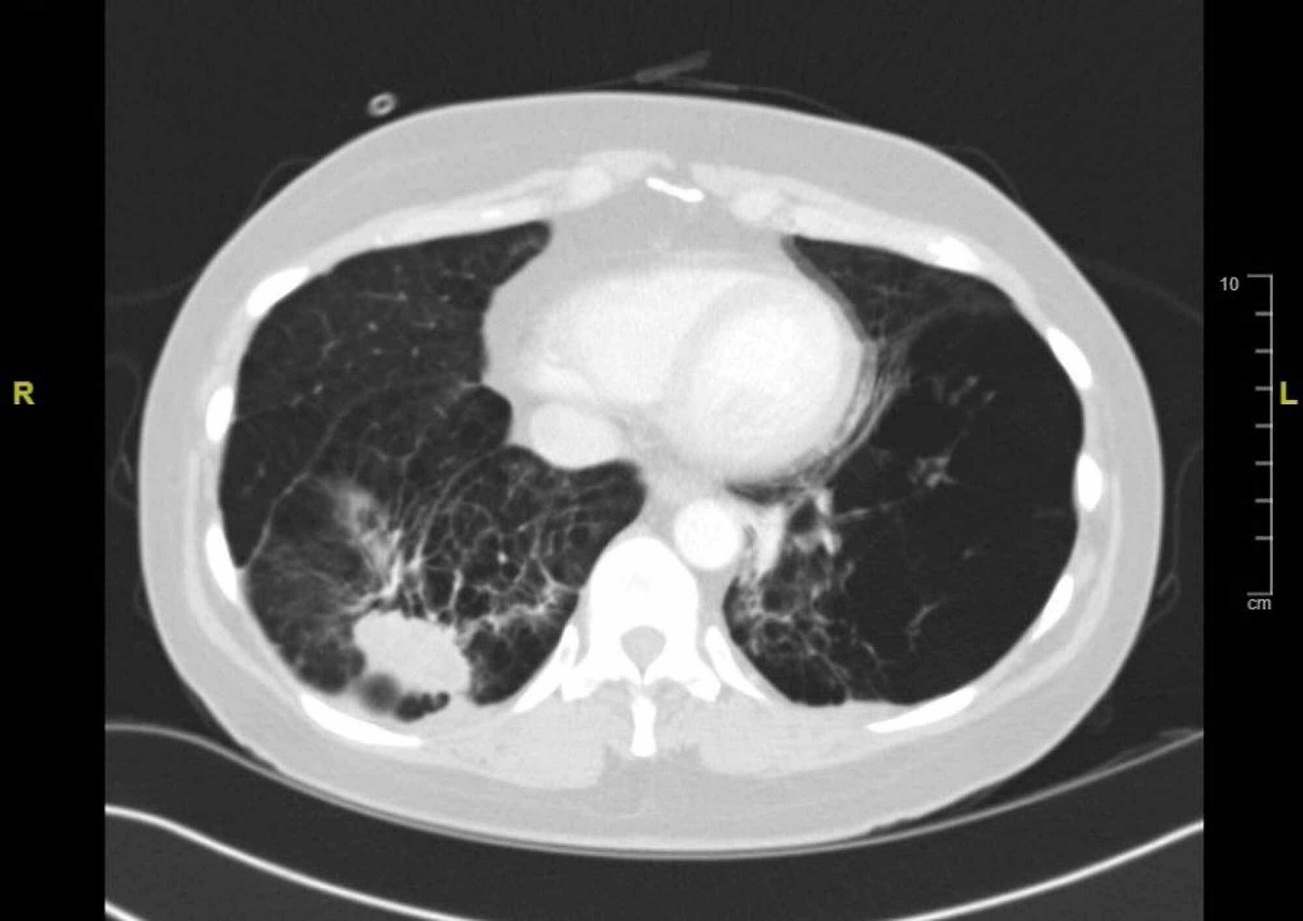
Initial CT of the chest Focal consolidation versus mass in the setting of emphysema is seen in the right lower lung base.
